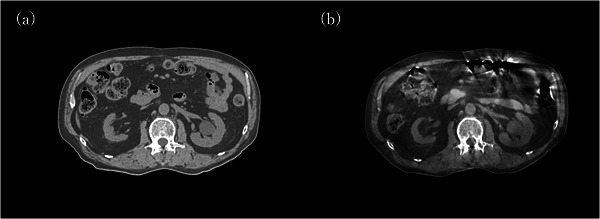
Representative images of daily CT and EP‐CBCT. (a)Daily CT, (b) EP‐CBCT. CT, computed tomography; EP‐CBCT, expiratory‐phase cone‐beam computed tomography.
